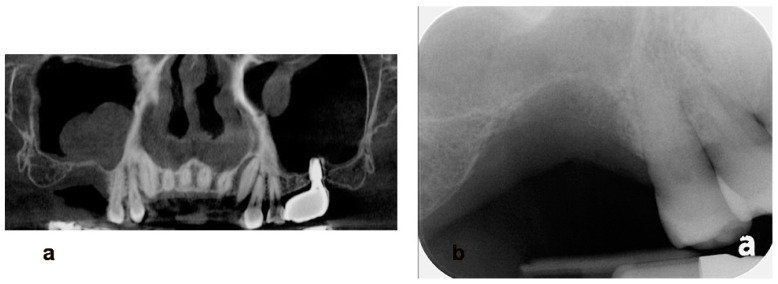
(a) Preoperative CBCT scans indicate a huge retention cyst on the right sinus. (b) A plain radiograph reveals 1 mm of residual bone height and an advanced ridge defect.
